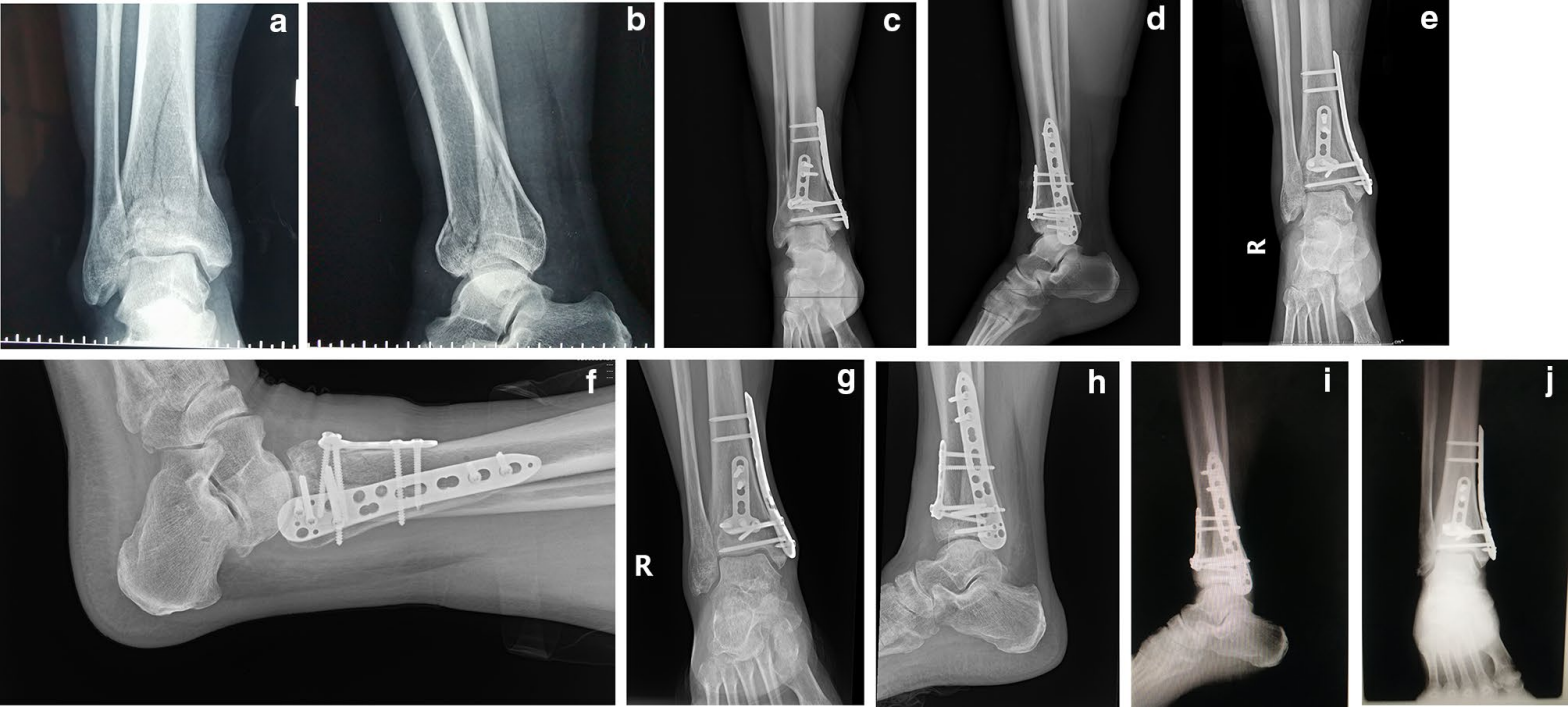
The anteroposterior and lateral X-rays of a pilon fractures. (a) and (b) Preoperative anteroposterior and lateral X-rays. (c) and (d) Anteroposterior and lateral X-rays on the day after surgery. (e) and (f) Anteroposterior and lateral X-rays 1 month after surgery. (g) and (h) Anteroposterior and lateral X-rays 3 month after surgery. (i) and (j) Anteroposterior and lateral X-rays 12 month after surgery.
